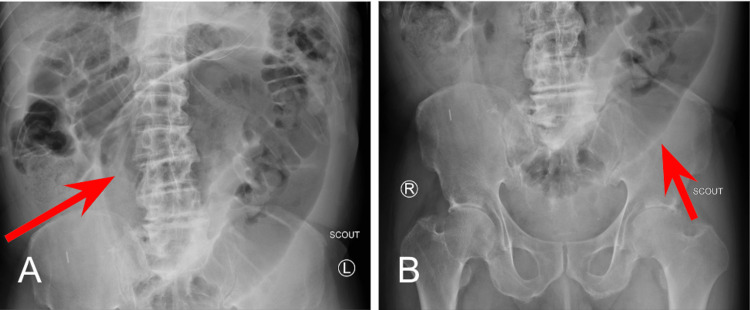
Plain Radiographs Prior Oral Contrast Administration 1A: Upper abdomen; 1B: Lower abdomen
